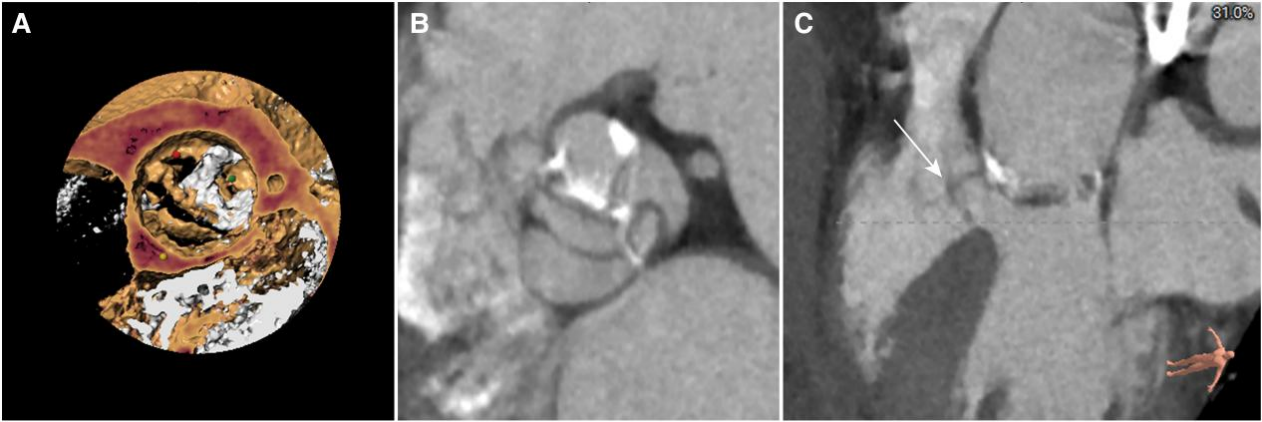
Multidetector computed tomography images of the aortic valve and septal aneurysm. (*A*) The type 1 bicuspid aortic valve in 3D view; (*B*) the type 1 bicuspid aortic valve with a septal aneurysm in the short view at a 7.2 mm height above the annulus; (*C*) the septal aneurysm originates from below the aortic annulus in the long view.
